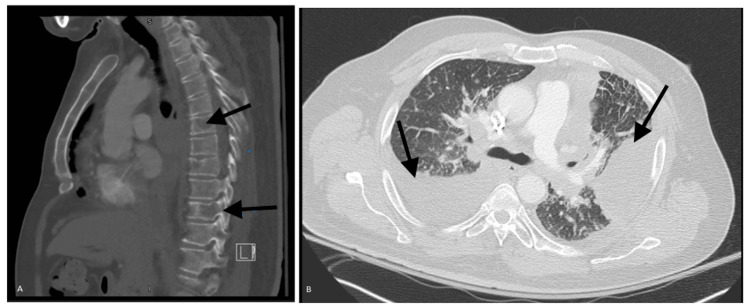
Follow-up CT scan showing disease progression A: Sagittal view of the thoracic spine demonstrating multiple lytic lesions, indicating areas of bone destruction typically seen in metastatic disease or primary bone pathology. The lesions appear as radiolucent areas within the vertebral bodies B: Lung metastasis with associated pleural effusion. The metastatic lesion is identified within the lung parenchyma, and the pleural effusion is noted as a fluid collection in the pleural space, likely secondary to the metastatic spread CT: computed tomography
